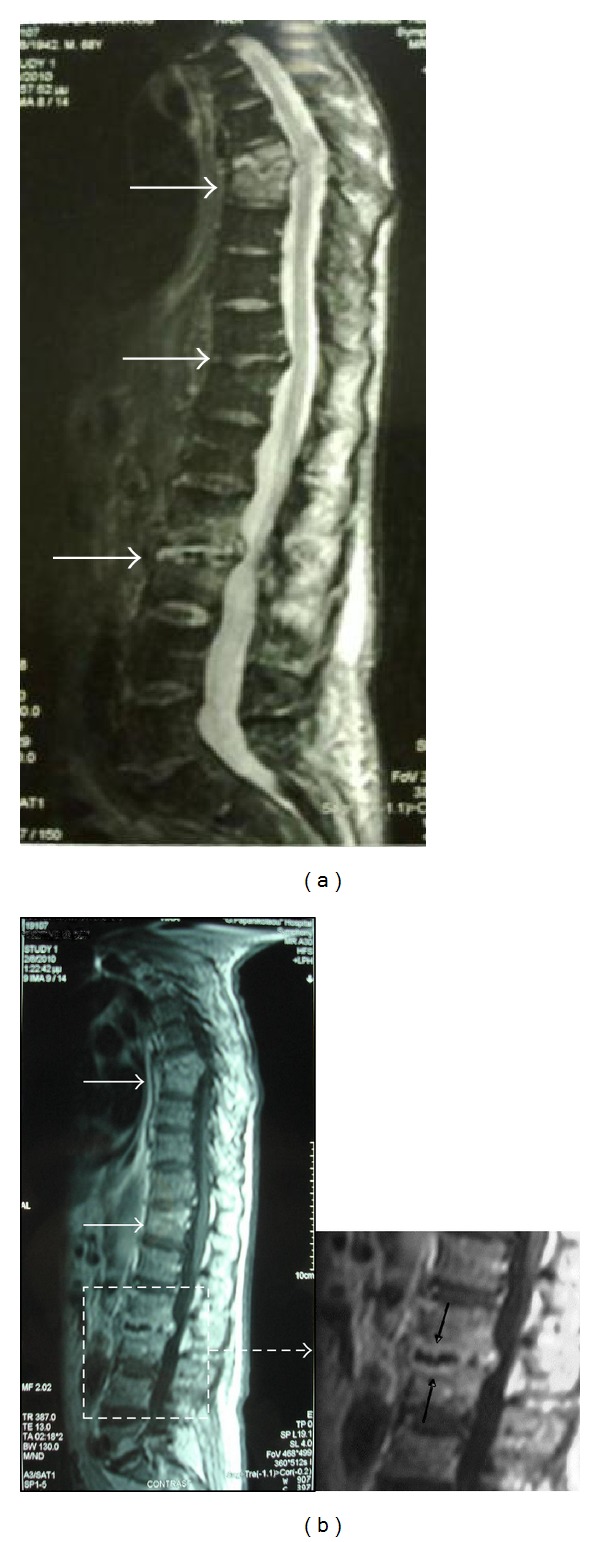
(a) Magnetic resonance imaging of thoracic and lumbar spine showing spondylodiscitis with a three-level involvement; T7-T8, T11-T12, and L2-L3 (white arrows). (b) A typical lesion is shown in the enlarged frame of the L2-L3 level; ellipsoidal contrast enhancement of the vertebral end-plates and the anterior aspect of the related disc space (black arrows).
